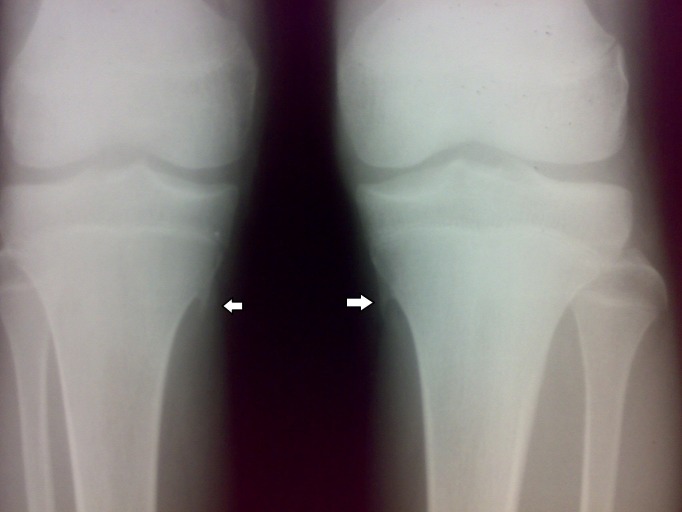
Case 1: Plain radiograph of bilateral knees—anteroposterior view Bilateral, symmetrical, sharp, bony spurs (white arrows) arising from the proximal tibial metaphysis corresponding to attachment of the hamstring tendons.
